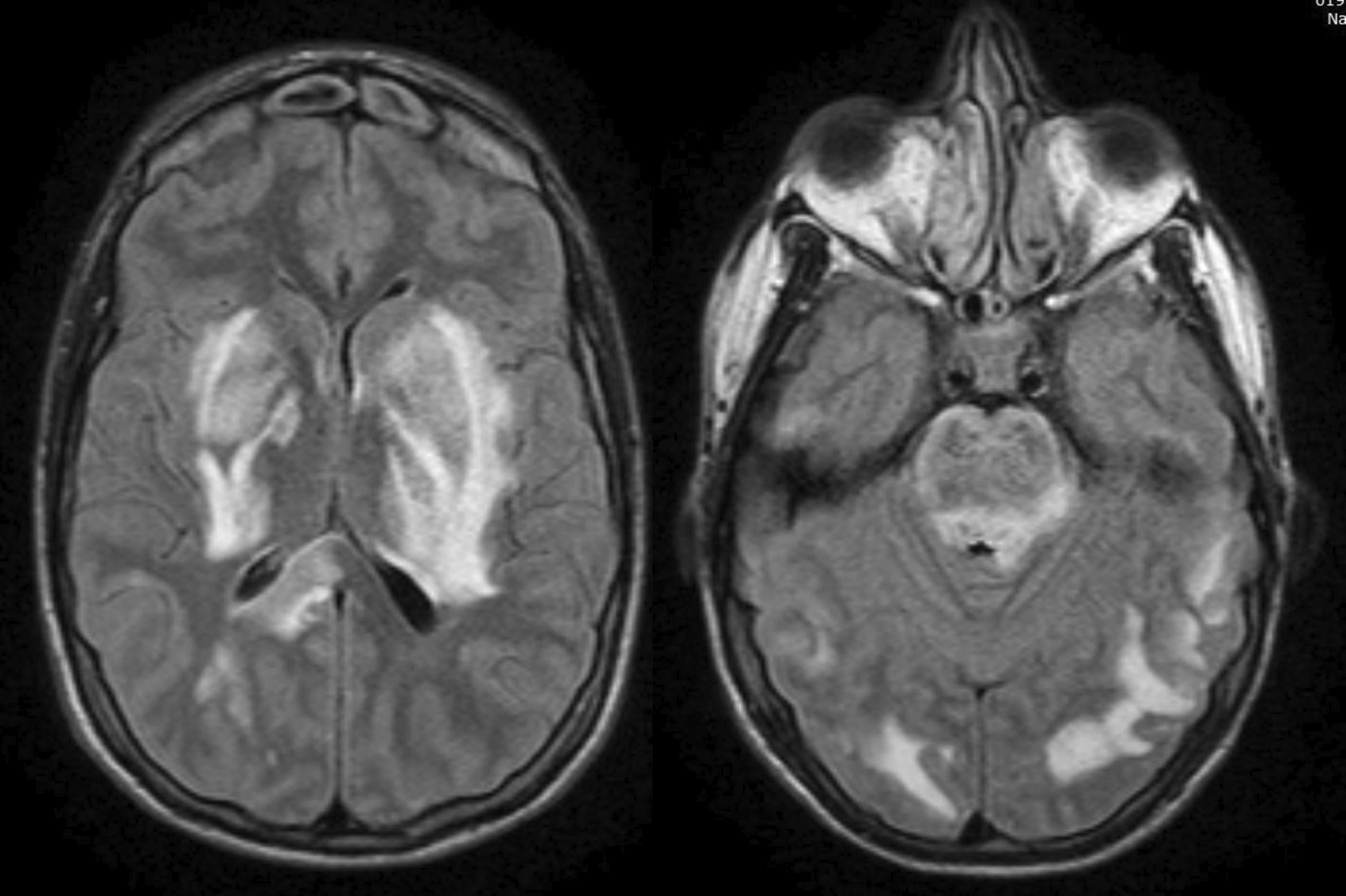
Brain Magnetic Resonance Imaging revealing extensive hyperintense lesions in T2 and FLAIR sequences, hypointense in T1, involving bilateral subcortical and periventricular posterior regions, with pontine medulla junction involvement compatible with vasogenic edema. The hyperintense rim that delimits the lentiform nucleus suggests the lentiform fork sign. No diffusion restriction was observed.
